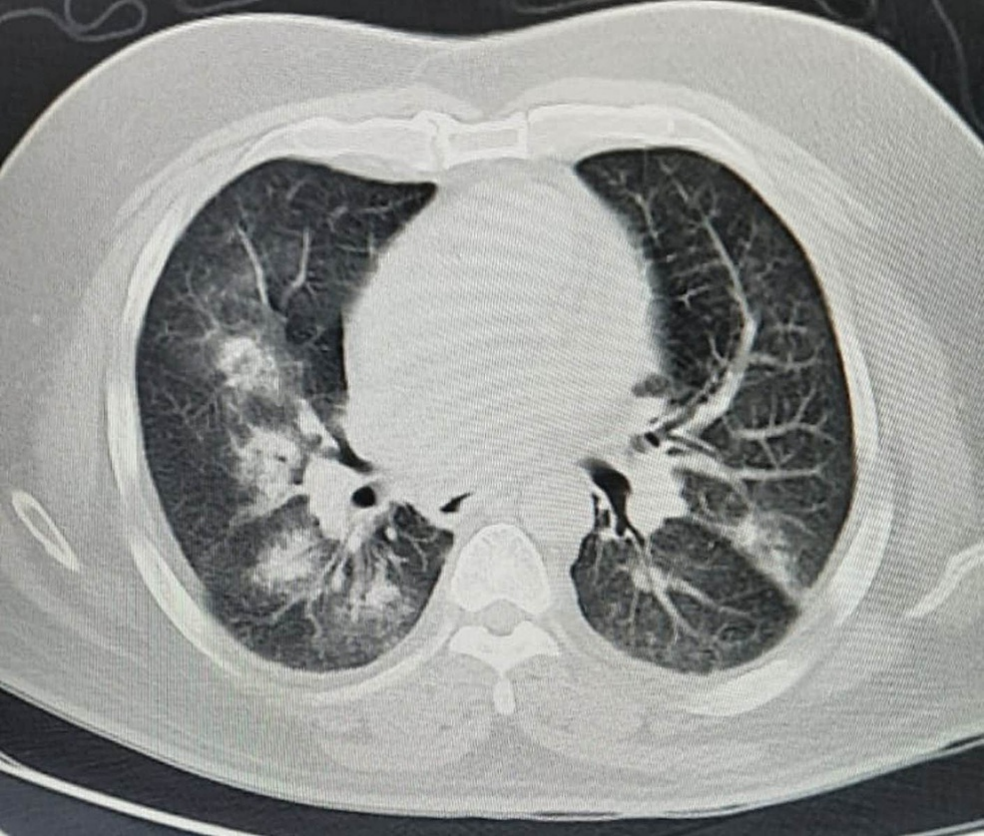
HRCT chest showed extensive bilateral ground-glass opacities HRCT: high-resolution computed tomography
